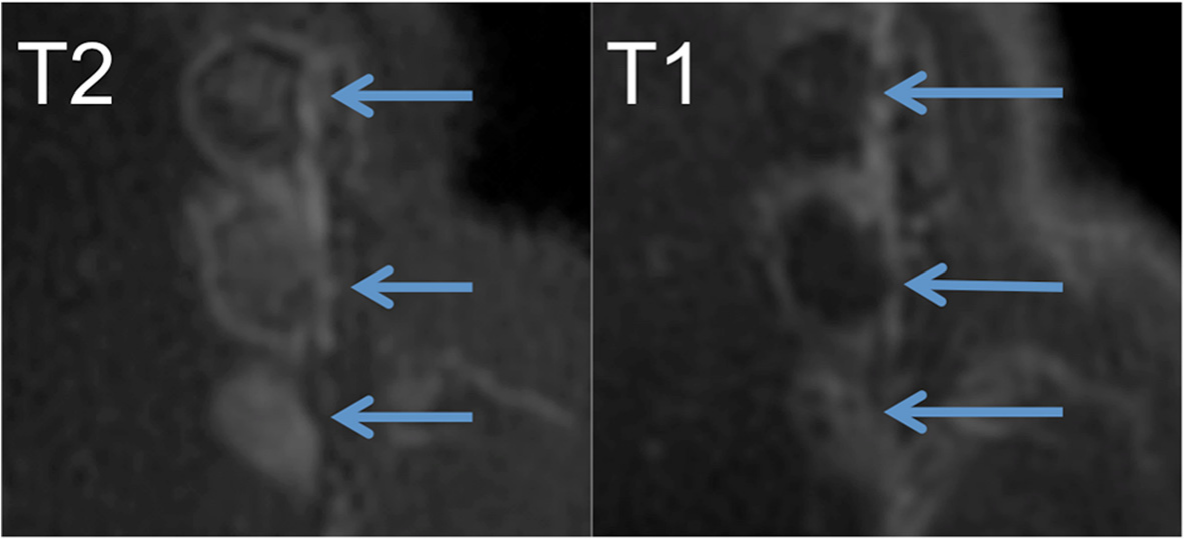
Sagittal MR images of the paraventricular muscles obtained on T2-FSE (*left*) and DCE T1-FSE. T2-FSE image shows the hyperintense edematous ablated lesion, while T1-FSE image shows the donut pattern enhancement after administration of Gd-DTPA
